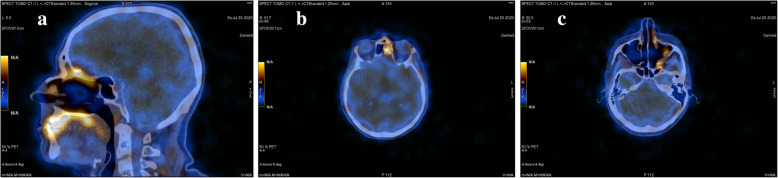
Post-surgery SPECT-CT somatostatin receptor scintigraphy scans showing slightly increased radiotracer uptake in the area of the left ethmoid region
